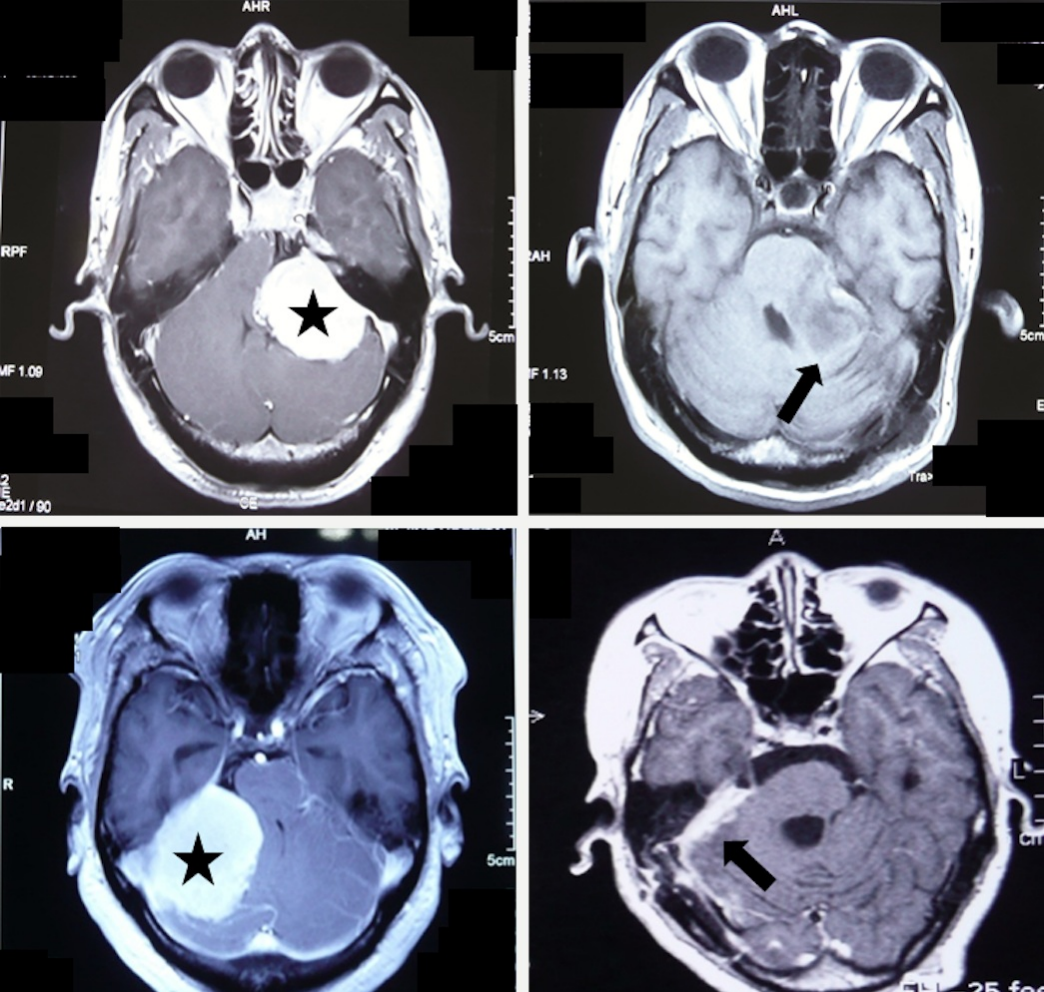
Total and Subtotal Resections MRI with contrast demonstrating the surgical removal of a CPA meningioma from two patients via a retrosigmoid approach. Upper row: a 36-year-old male who had total removal of his tumor (star). Lower row: a 48-year-old female with near total removal of her tumor (star). A small residual was left at the tumor origin at the back surface of the petrous bone. CPA: cerebellopontine angle; MRI: magnetic resonance imaging
